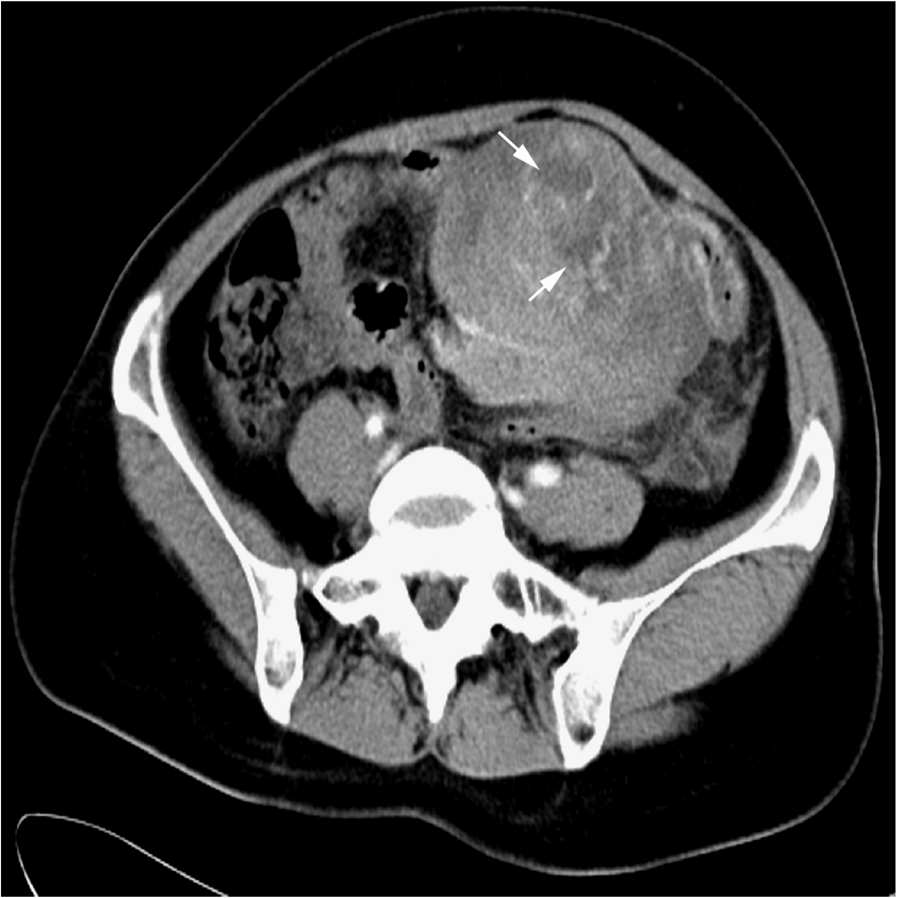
Preoperative computed tomography (CT) scan of the mesenteric mass. Contrast-enhanced CT demonstrating a poorly circumscribed solid mass with mild heterogeneous enhancement located at mesentery and showed adhesions of wall of jejunum. The multiple irregular hypointensity areas were observed in the mass and considered to be necrotic areas (white arrow).
